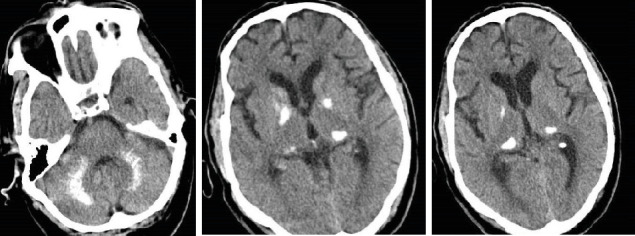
Noncontrast axial computed tomography image shows bilateral calcification of basal ganglia, pulvinar region of thalami, and dentate nuclei.
